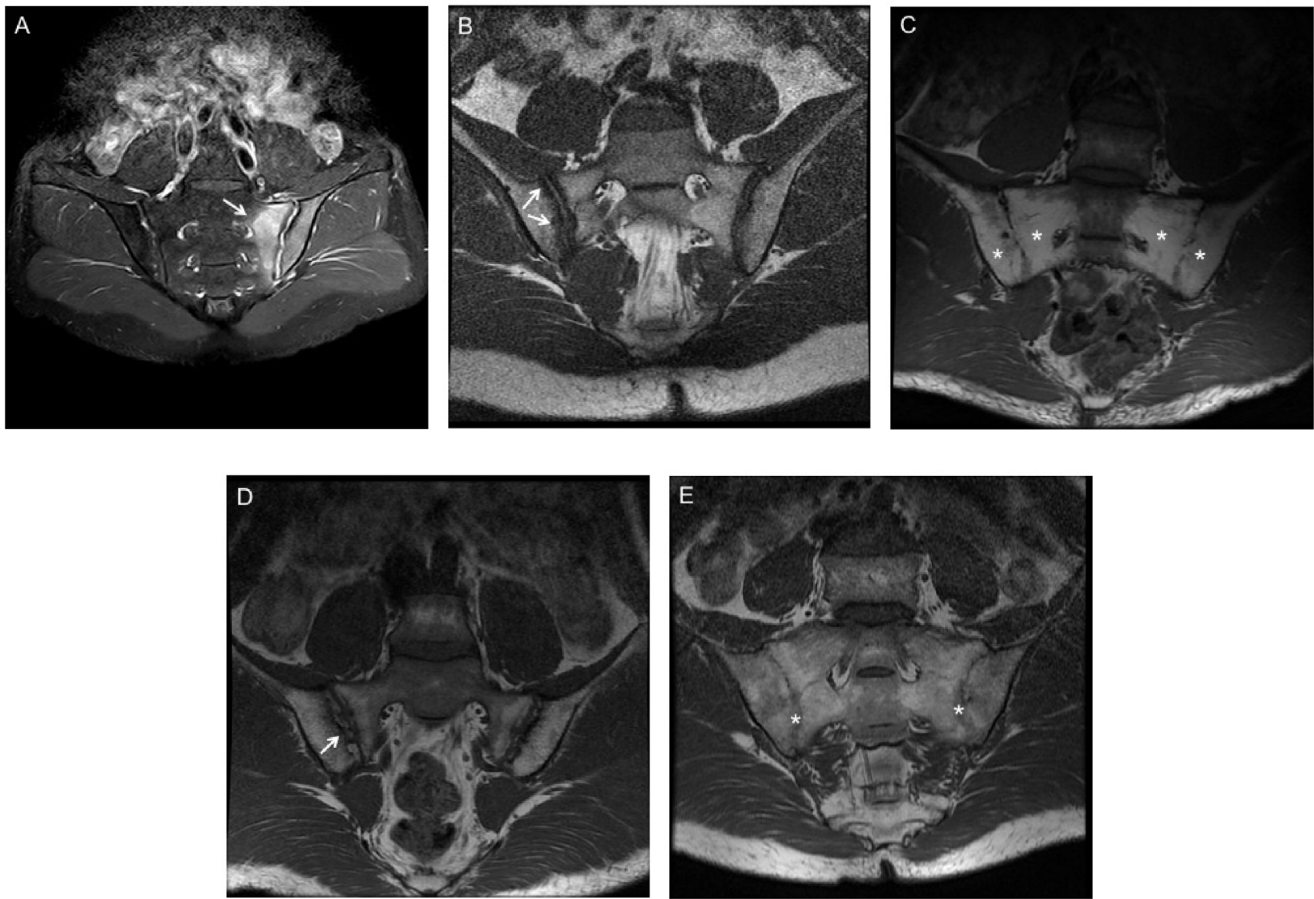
MRI findings of inflammatory and structural damage of SpA. (A) Bone marrow edema (BME) at the left side of sacroiliac joints (white arrows) in Coronal oblique STIR sequence. (B) Erosion of the right iliac bone on T1 weighted spin echo MRI (white arrows). (C) Fat metaplasia at the bilateral sacroiliac joints on T1 weighted spin echo MRI (white stars). (D) Backfill of the right iliac bone on T1 weighted spin echo MRI (white arrows). (E) Ankylosis of the bilateral sacroiliac joints on T1 weighted spin echo MRI (white stars).
